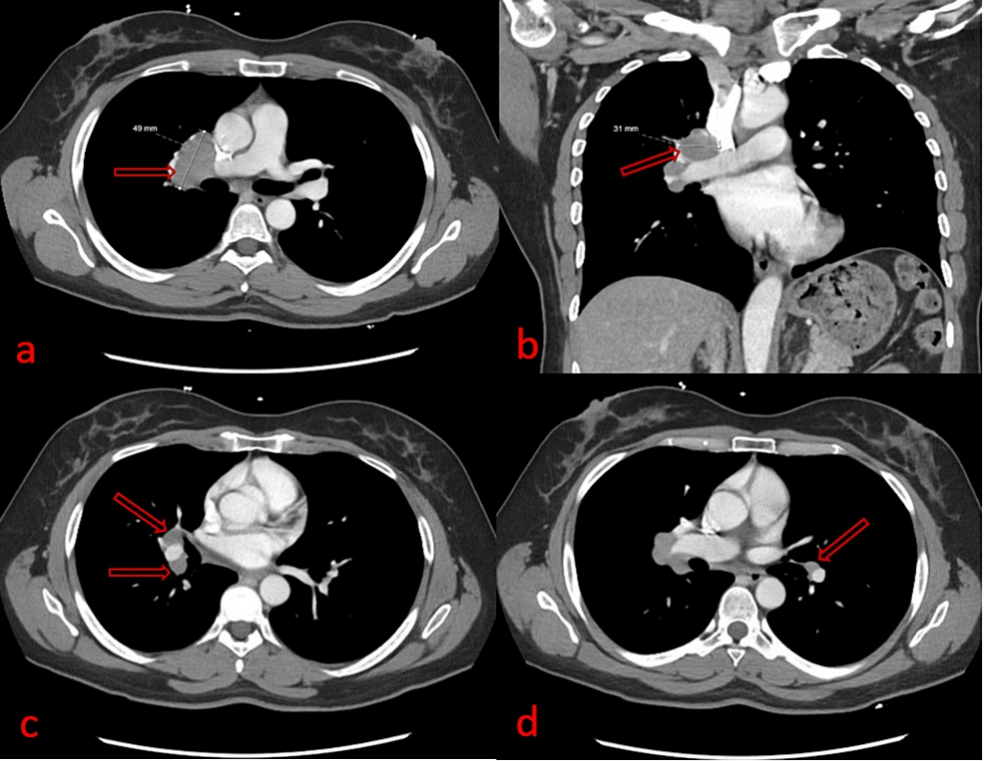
Chest axial (a, c, and d) and coronal (b) computed tomography images (CT) with contrast showing multiple enlarged right hilar lymph nodes (a, b, and c) and mildly enlarged left hilar lymph node (d). The largest enlarged right hilar lymph node measures 4.9 x 3.1 cm (a and b).
